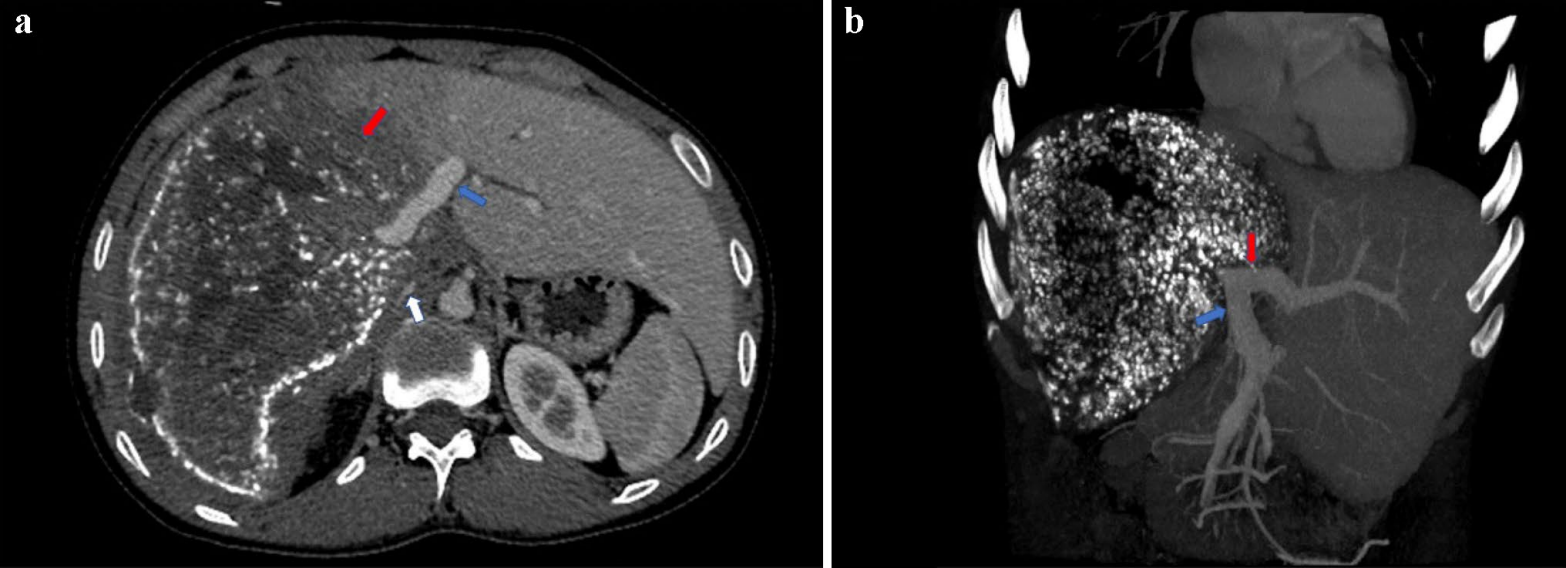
A 31-year-old female patient with HAE. a Axial CT image in the portal venous phase showed that the HAE lesion (red arrow) contacted the LPV (blue arrow), with the presence of an irregular wall and lumen stenosis. The RPV did not show due to lumen occlusion. The lesion-vessel contact angle of IVC (white arrow) was grade 5 accompanied by an irregular wall and lumen stenosis. b MIP image showed occlusion of the RPV, LPV (red arrow) showed with irregular wall and lumen stenosis, MPV (blue arrow) showed a little lumen stenosis. Pathological results confirmed that the LPV, RPV and IVC were all invaded by HAE, but MPV was normal. LPV, left portal vein; RPV, right portal vein; MPV, main portal vein; IVC, inferior vena cava; MIP, maximum density projection
